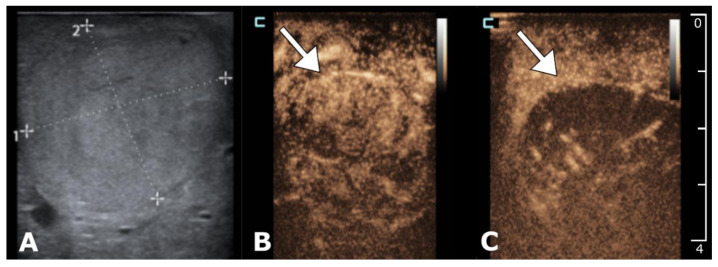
Intraoperative contrast-enhanced ultrasound (US) for tumor identification and treatment evaluation. B-mode ultrasound showing 24 mm subcapsular HCC in liver segment III (A). Contrast-enhanced ultrasound (CEUS) prior to ECT demonstrating a hypervascular tumor with early enhancement (B). CEUS two minutes post-ECT demonstrates reduced enhancement of the tumor with contrast due to the vascular lock effect (C).
